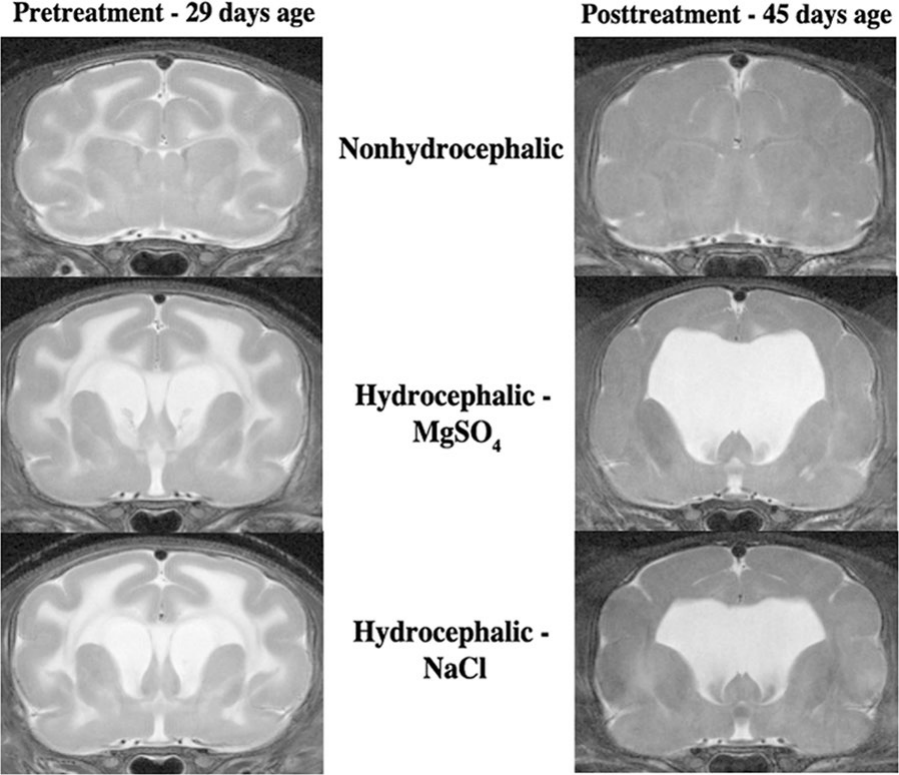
T2-weighted magnetic resonance images showing frontal coronal slices of brains of ferrets without hydrocephalus as well as ferrets that were treated with MgSO_4_ or NaCl between 29 and 45 days age. Progressive ventriculomegaly is evident in these examples of both treatments
